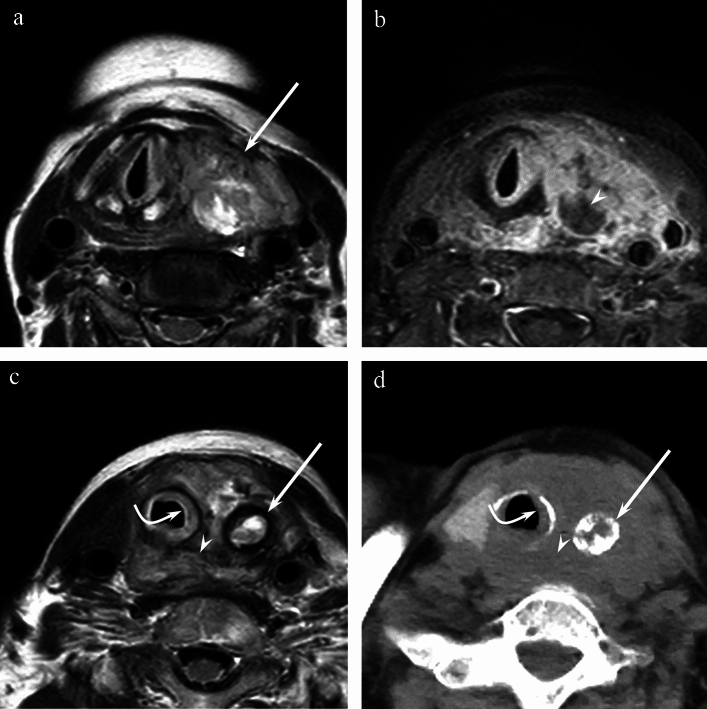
A 79-year-old woman with anaplastic thyroid carcinoma of the left lobe. a T2-weighted image (TR/TE, 4620/100 ms) shows a heterogeneously hyperintense mass with an ill-defined margin (arrow). b Fat-suppressed contrast-enhanced T1-weighted image (TR/TE, 656/18 ms) shows heterogeneous enhancement with unenhanced areas (arrowhead). c T2-weighted image shows intratumoral ring-shaped hypointensity (arrow). Tracheal (curved arrow) and esophageal (arrowhead) invasions are demonstrated. d Unenhanced CT image shows intratumoral ring-shaped calcification (arrow) corresponding to the ring-shaped hypointensity on T2WI. The tumor shows an ill-defined margin, tracheal invasion (curved arrow), and esophageal invasion (arrowhead)
